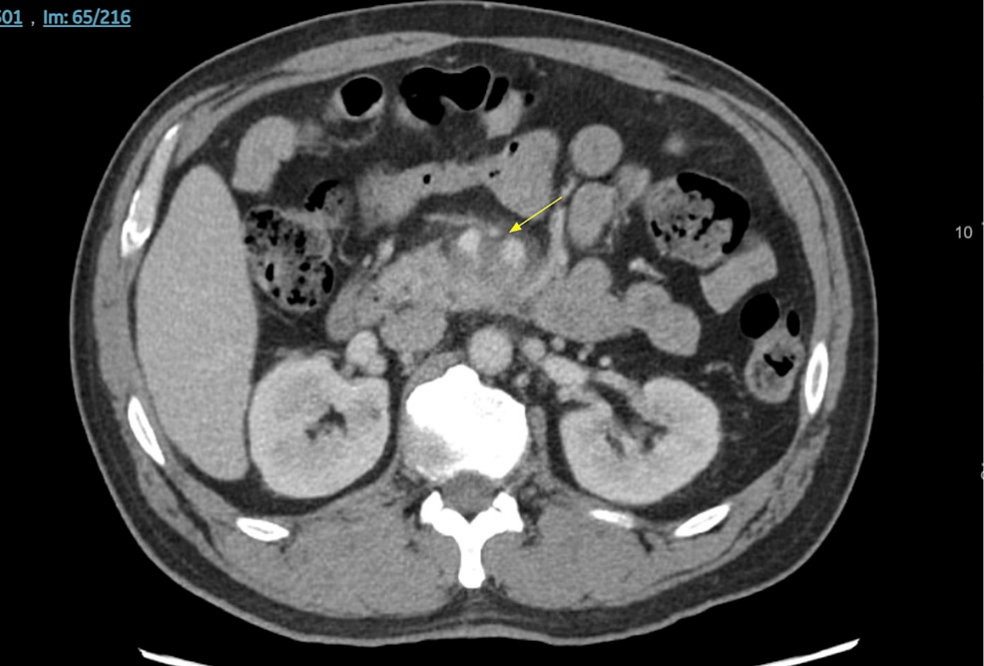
A CT scan of the abdomen and pelvis with contrast shows occlusive thrombosis involving the proximal jejunal vein. A hazy inflammatory stranding was noted abutting the pancreatic uncinate process, which raised concern for focal acute pancreatitis or infiltrative pancreatic neoplasm. The yellow arrow points at where the proximal jejunal vein abruptly stops before draining into the superior mesenteric vein. Directly posterior to this point, the previously mentioned hazy inflammatory stranding is visualized.
